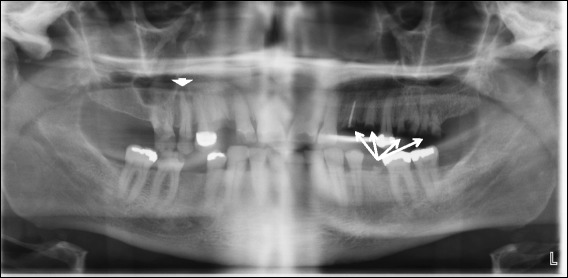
Dental panoramic radiograph demonstrated multiple missing teeth and dental amalgam due to prior dental caries. Most notably there were numerous erosions of the crowns (*arrows*) of many teeth with a mottled appearance of the residual root and periapical lucencies (*arrowhead*). These carious nonrestorable teeth were later extracted
